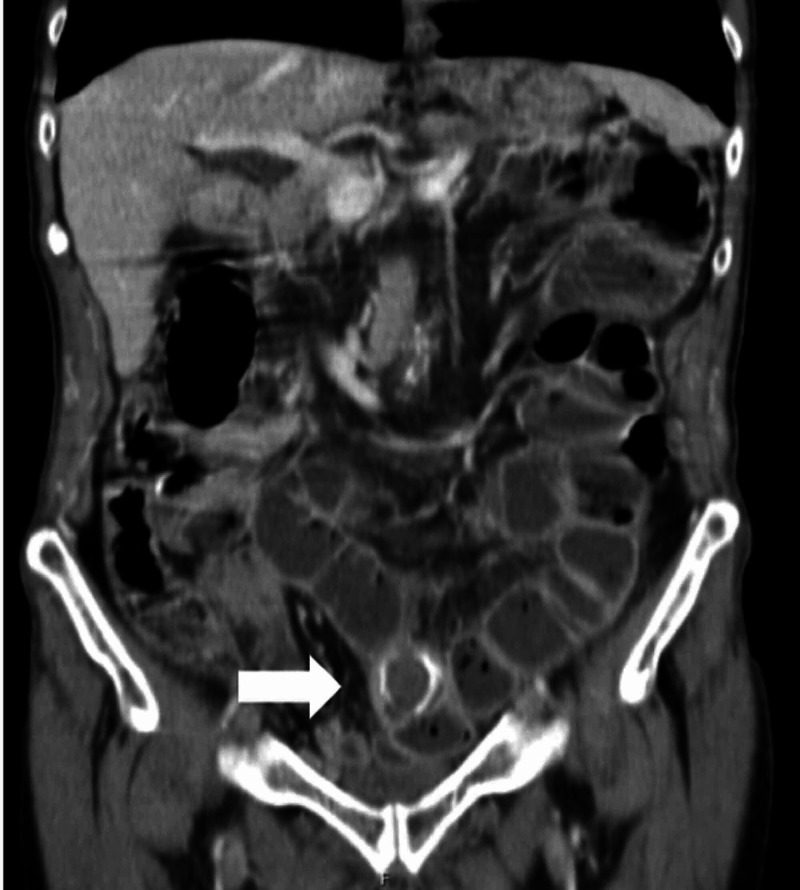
Abdomino-pelvic CT (coronal section) revealing the gallstone (white arrow) impacted in terminal ileum, causing proximal small bowel dilatation.
